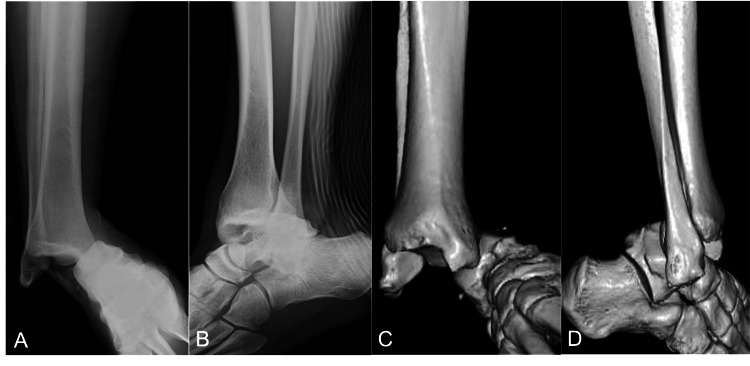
Preoperative plain radiographs and CT scans of the patient's right ankle. Plain anteroposterior (A) and lateral (B) radiographs revealed dislocation. The 3D-CT scans (C, D) showed dislocation without any associated fracture.
